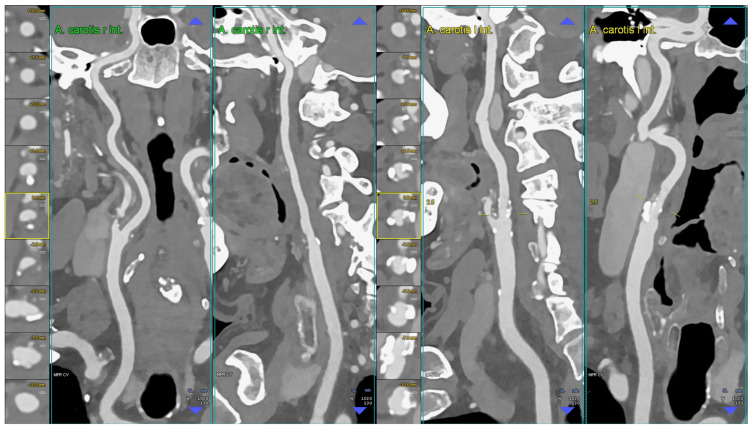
CT angiography of the carotid arteries; moderate bilateral extra-cranial atherosclerotic disease. The figure shows a CT angiography of the carotid arteries performed using a whole body PCCT scanner but using reconstruction parameters comparable to the best ones applicable by a state-of-the-art non-PCCT scanner (in summary: 0.6 mm slice thickness with 0.4 mm slice increment, medium convolution kernel); in particular, the figure shows orthogonal longitudinal multiplanar reconstruction of the right and left carotid artery axes with corresponding series of axial cross-sections of the respective bifurcation on left of each one. The image shows mild predominantly non-calcified atherosclerosis on the right bifurcation and mild predominantly calcified atherosclerosis on the left bifurcation. Abbreviations: CT = computed tomography; PCCT = photon-counting CT.
